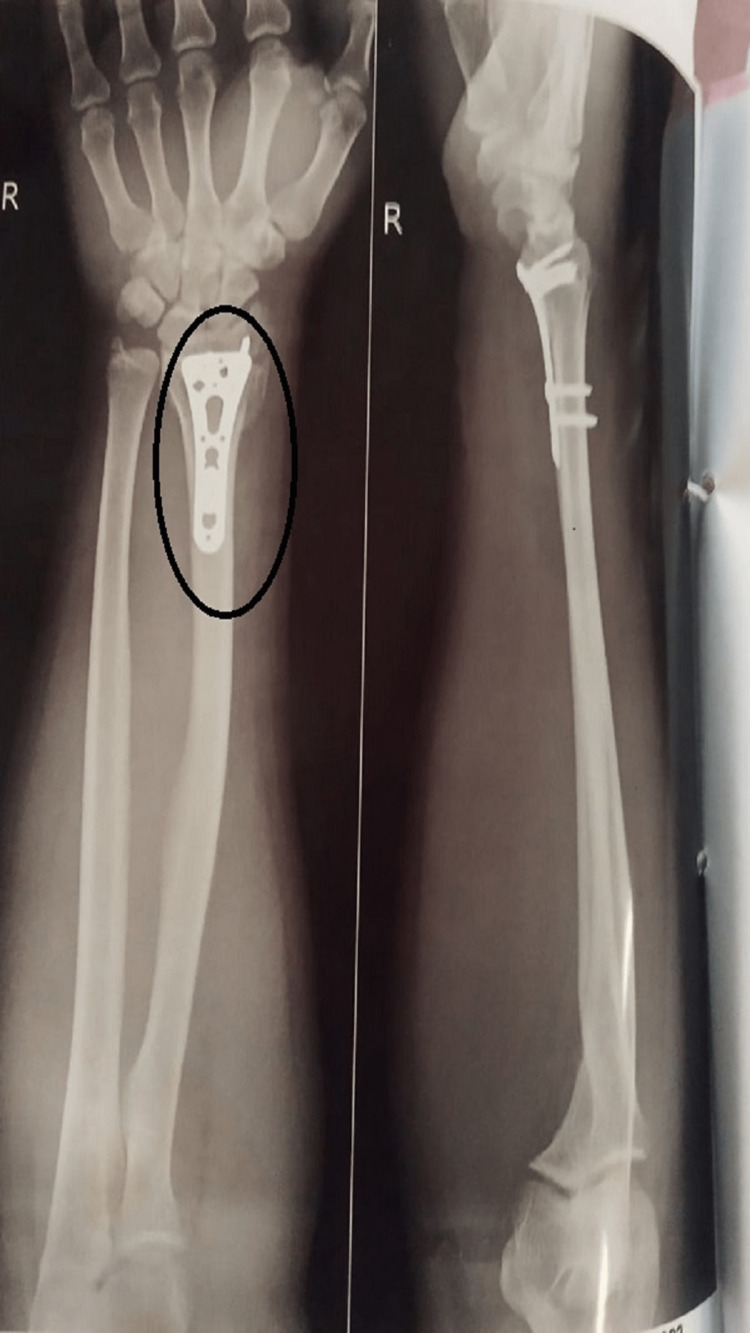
Post-operative X-ray of the wrist
